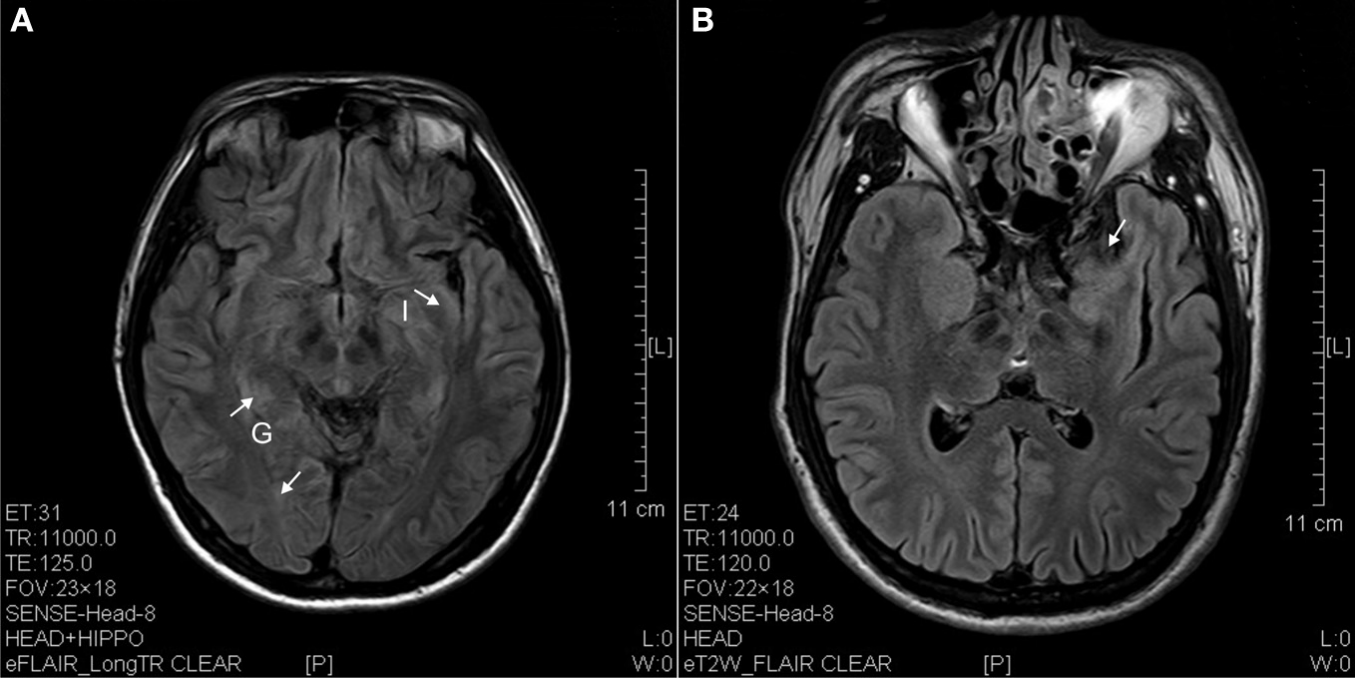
FLAIR image of diffusion blockage as hyperintense spots in the parahippocampal gyrus (G) and insula (I) as well as atrophy of uncus (arrow). (A) Ketamine addict of 4 years. (B) Ketamine addict of 5 years.
